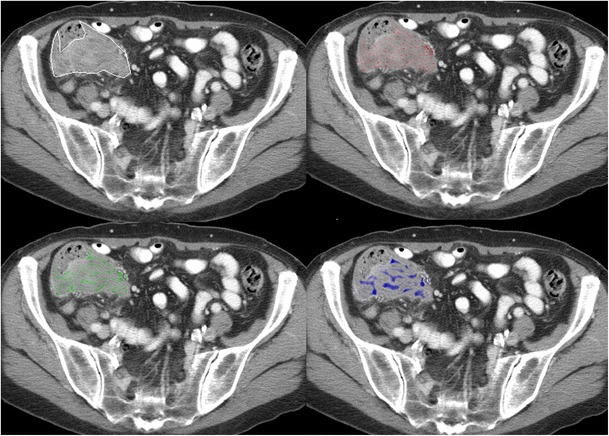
Texture analysis of contrast-enhanced CT images of a colon cancer with the application of different filters highlighting fine, medium, and coarse textures
